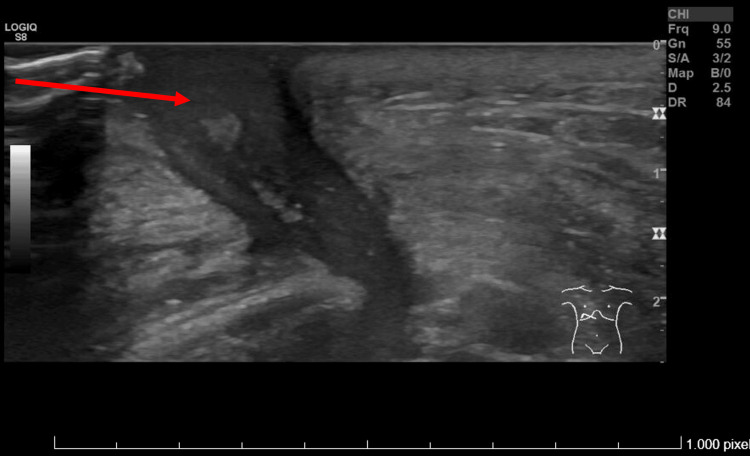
Chest ultrasound image The red arrow shows the chest wall mass.
